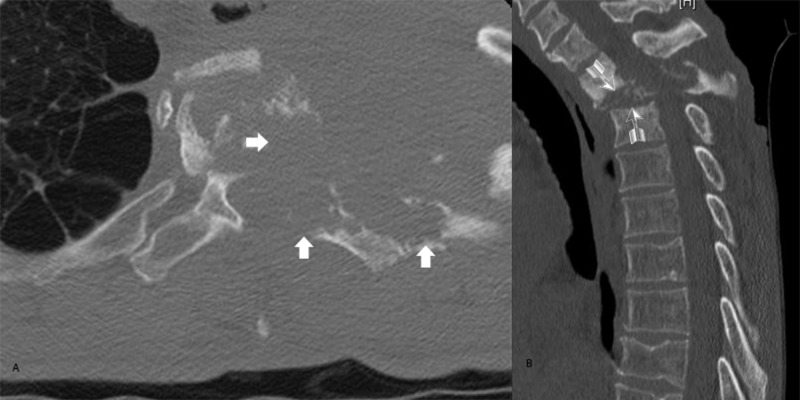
Pre-Operative (A) Axial and (B) Sagittal Bone Windowed CT Scan Thick Arrows: Tumor Invasion of bone in the vertebral body, posterior elements, and rib head. Feathered Arrows: Kyphotic Deformity
